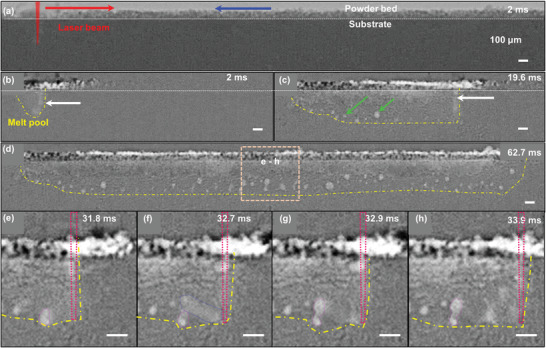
Time‐series radiographs show the formation of melt track and pores during LPBF of Inconel 625 (*P* = 150 W, *v* = 50 mm s^−1^, *t* = 100 µm, *d* = 50 µm, and SE = 0.095 MJ m^−1^ s^−1/2^). a) A radiograph before background subtraction (BGS) wherein light grey, medium grey and jet‐black colors show argon/background, Inconel 625 powder and substrate, respectively. The red arrow indicates the direction of the scanning laser beam, the blue arrow indicates the argon gas flow direction, and the yellow dotted line indicates the melt region. Selected BGS images reveal the pore formation phenomena at b) 2, c) 19.6, and d) 62.7 ms with a region of interest. The magnified images (e–h) show the formation of irregular pores where f) a gas pore is entrained by the Marangoni convection, g) merges with a pre‐existing pore, and h) retains its shape and position after solidification. Scale bar 100 µm. See details in Movie S1, Supporting Information.
